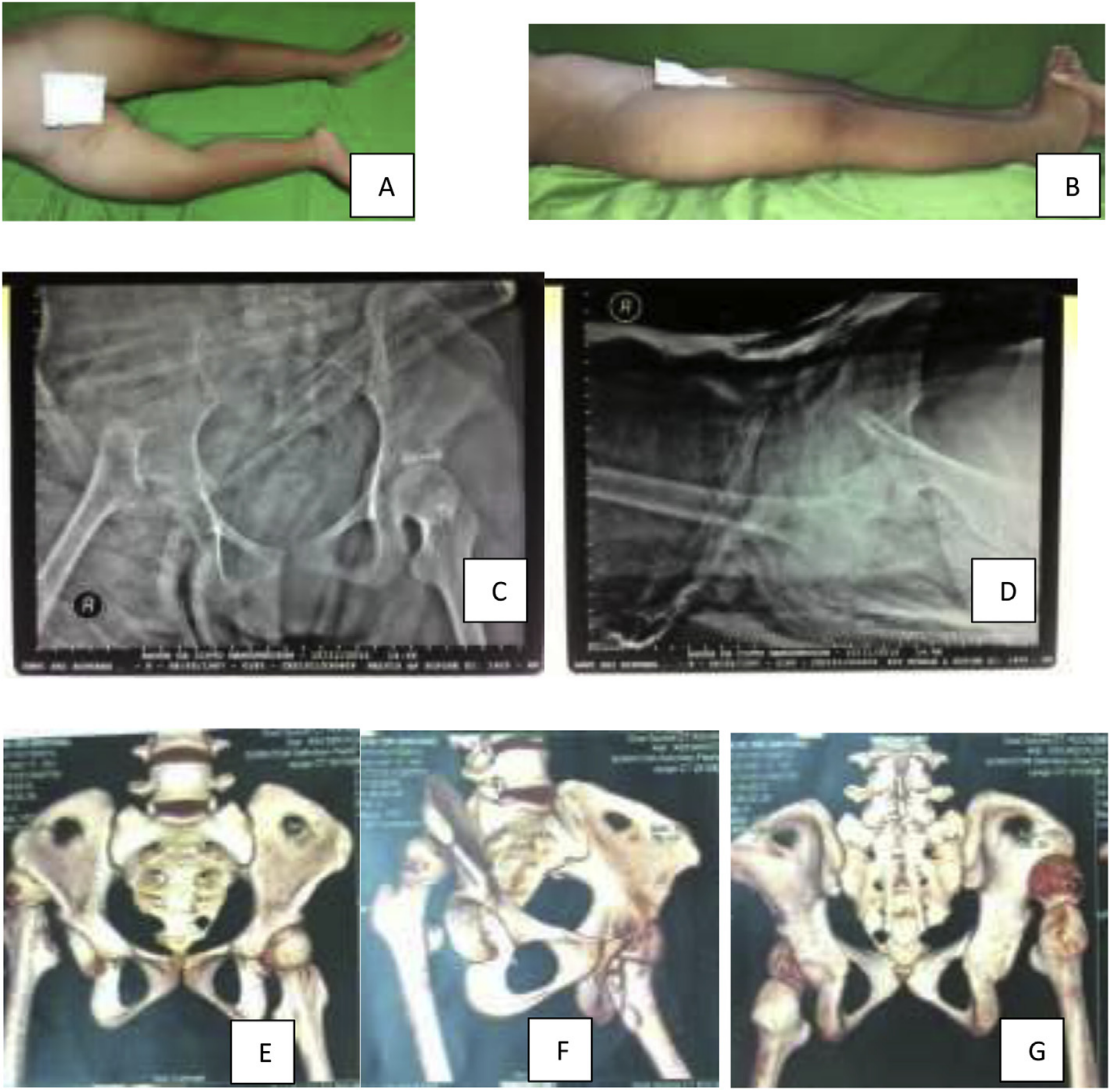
A-B showed clinical examination of patient. Female, a 5 cm leg length discrepancy was noted. C-D showed Pelvic AP and axial Xray. Radiograph of failed closed reduction and spica application showing a persistent dislocation. Acetabular index of right hip were 55^0^ and epiphyseal plate has closed. E-G showed Pelvic CT 3D reconstruction. Right posterior hip dislocation, with no concomittant fracture in acetabulum nor femoral head, shape of femoral head was still round.
